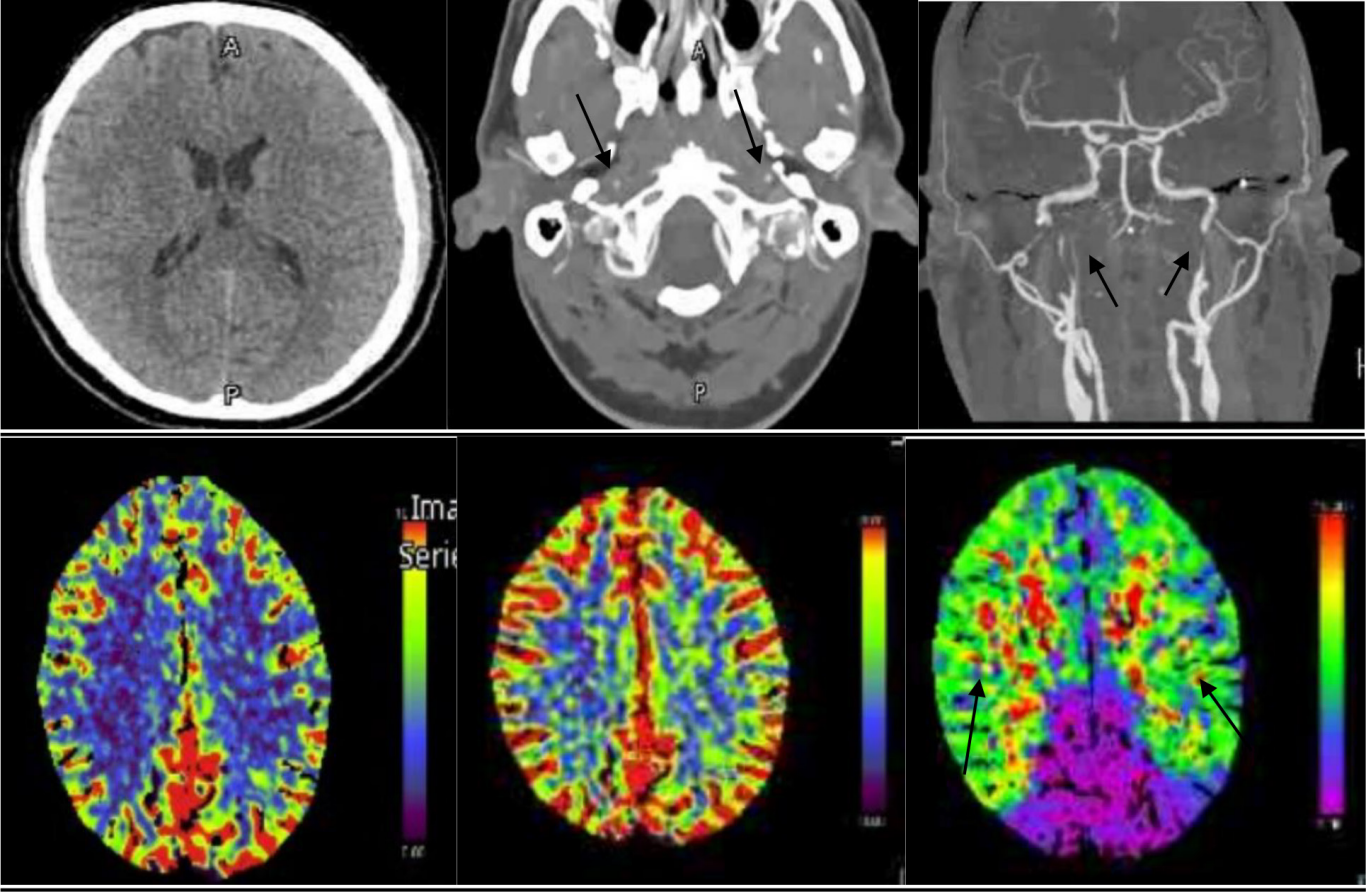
CT head showed normal brain parenchyma attenuation and no territorial hypodensity. CT perfusion showed symmetrical bilateral perfusion abnormality involving the anterior circulation with increased time to drain (TTD), likely secondary to severe stenosis of the distal cervical segments of the ICAs bilaterally. CT angiogram showed bilateral severe stenosis noted at the distal cervical segment of the bilateral ICAs and moderate stenosis at the V2 segment of the right vertebral artery.
